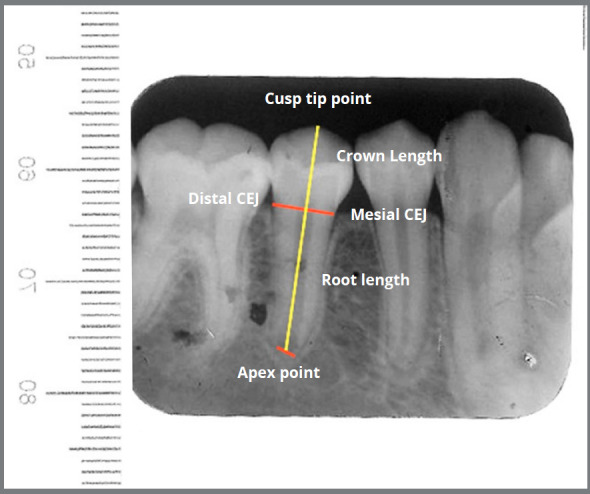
Measurement of crown and root length on digital scanned periapical radiographs with a transparent ruler to calibrate the image (production of pixel to millimeter conversion factor, PMCF).
